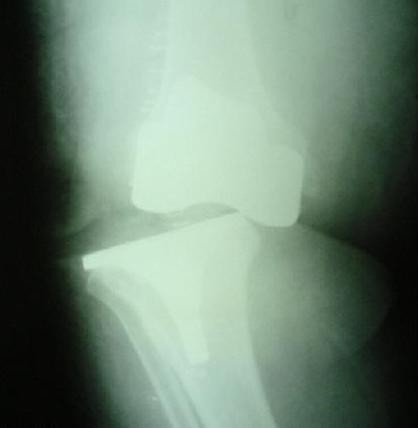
AP roentgenography of the knee at postoperative second day.
